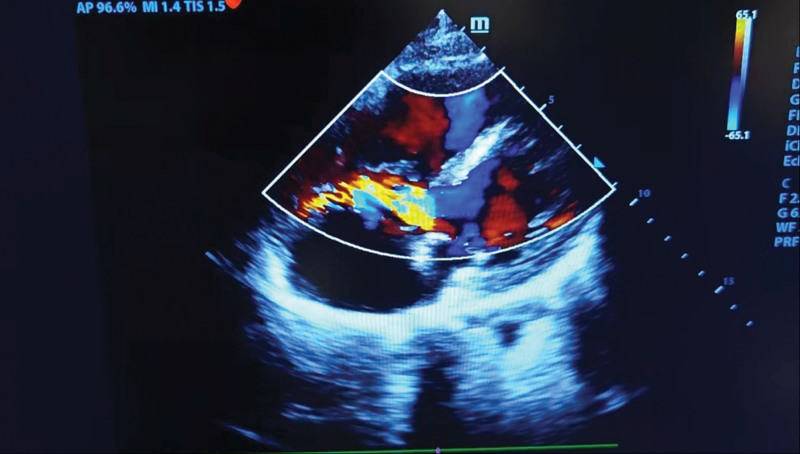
Transesophageal echocardiography with color Doppler showing a high-velocity multicolored (aliasing) mosaic of blood flow through the base of the sinus tumor.
